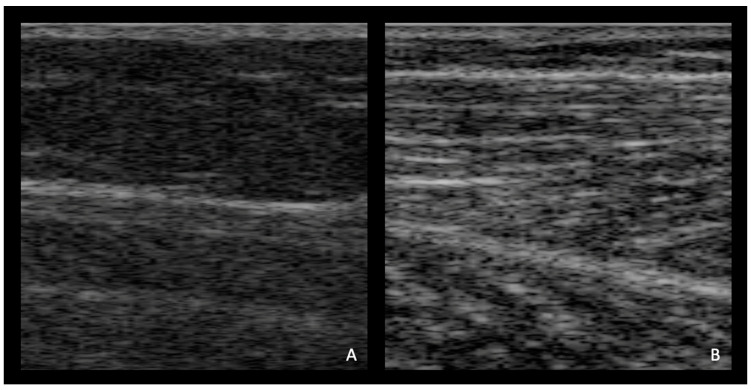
Randomly selected representative ultrasound images for (A) older adults and (B) young adults at 0.75 m/s 0.0° level ground.
